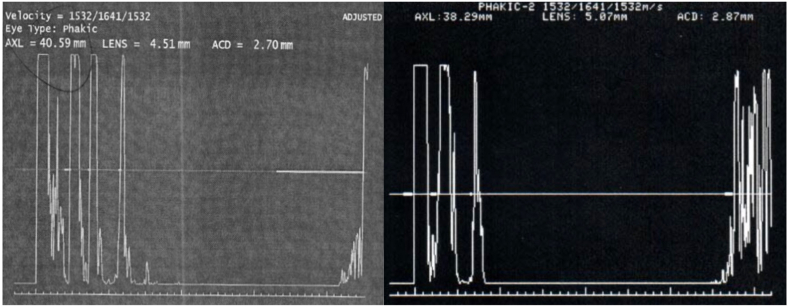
Preoperative immersion A scan ultrasound taken by the Eye Cubed V3 (Ellex, Minneapolis, Minnesota). Axial lengths measurements were 40.59 mm for the right eye by immersion and 38.29 mm for the left eye by applanation.
